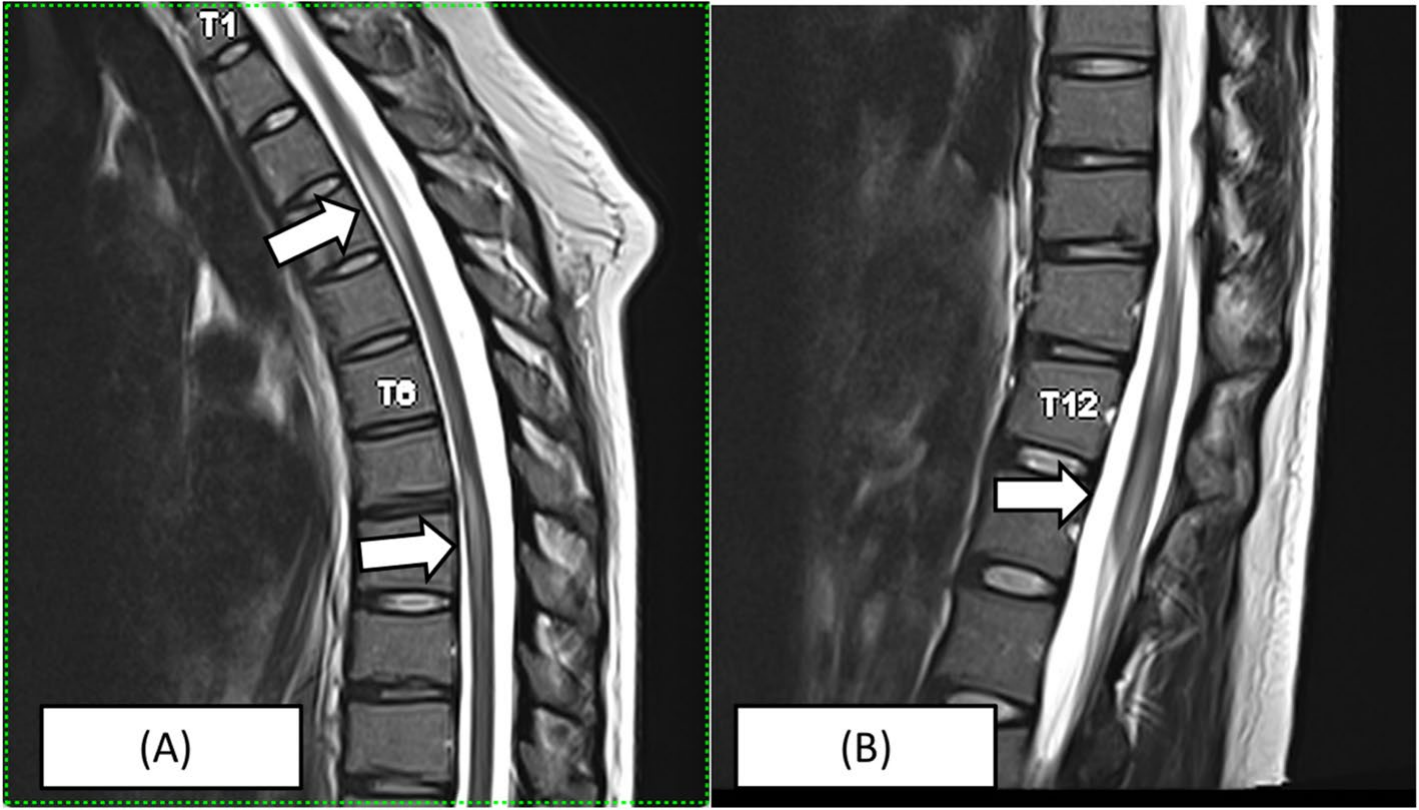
MRI spine of the patient. A T2 sagittal view showing expansile hyperintense lesion at the T3 – T5 level and T7 – T9 level. B T2 sagittal view showing slight central hyperintense lesion at T12 – L1 level
